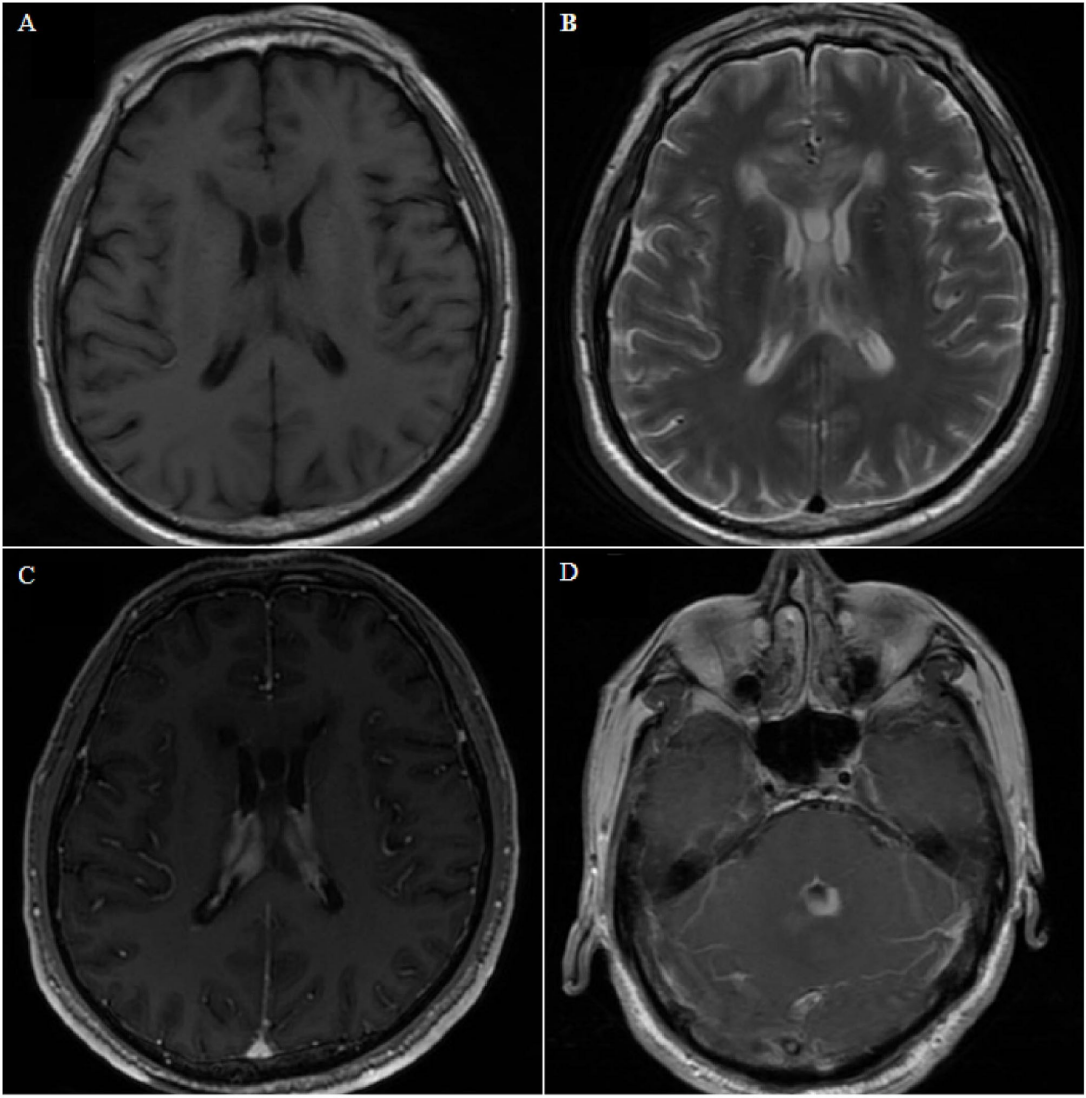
Case 8. Axial magnetic resonance image showing bilateral and fourth ventricle lesions, diffuse growth along the choroid plexus and ventricle wall, low signal on T1- and T2-weighted images (A,B), and homogeneous enhancement on contrast-enhanced T1-weighted images (C,D).
